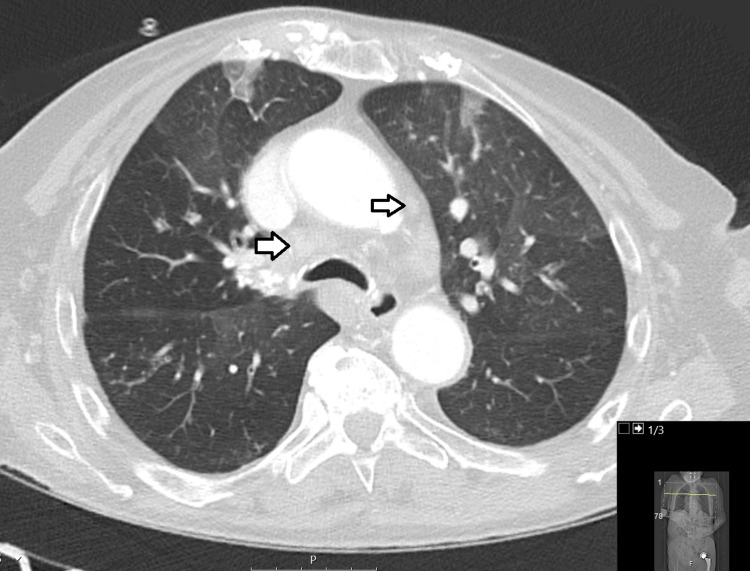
CT scan of the chest showing hilar lymphadenopathies.
